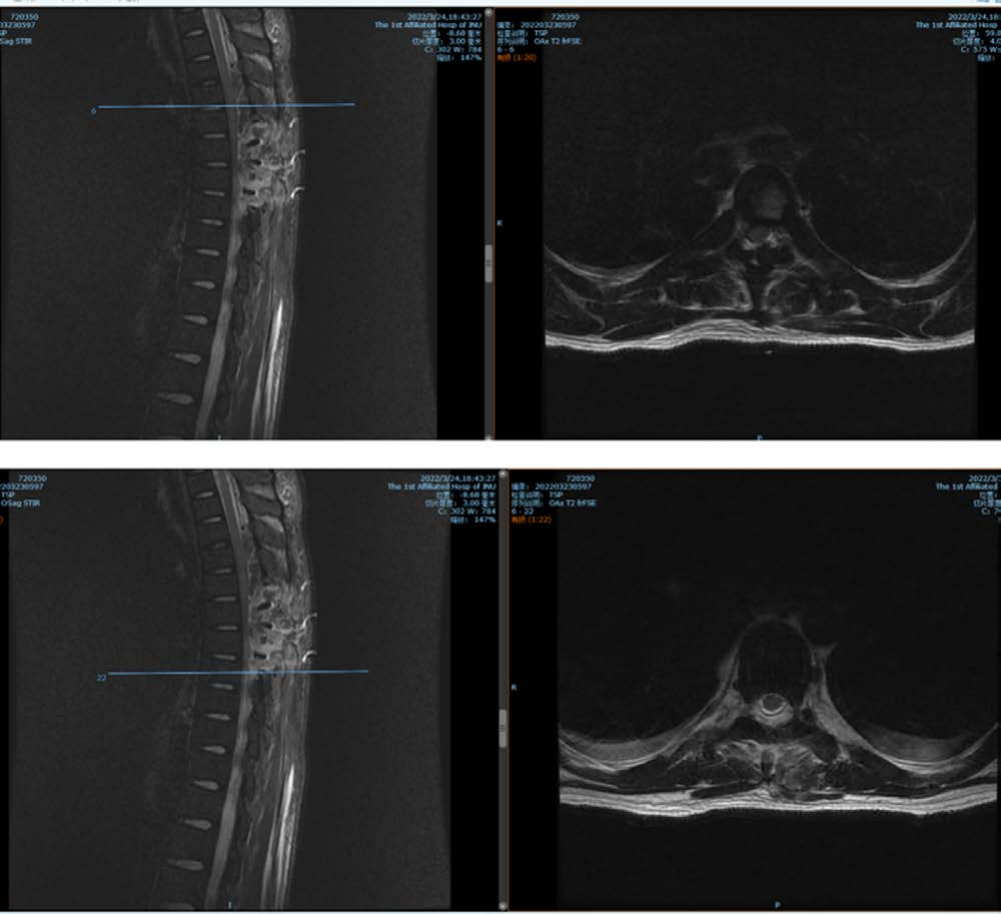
(MRI T2WI) Preoperative computed tomography (CT) and MR showing thoracic spine, rib bone abnormalities, spinal epidural space at the T4-T9 level, left T8 vertebral body, bilateral, multiple space-occupying lesions in the T9 vertebral body. MRI = magnetic resonance imaging.
